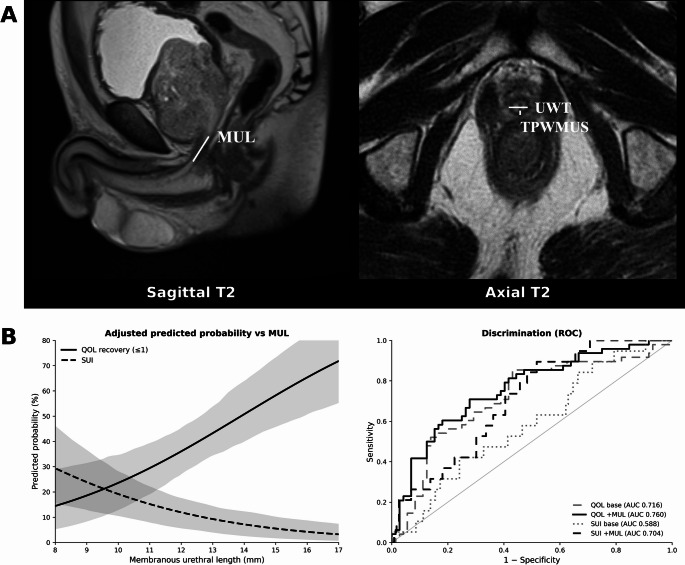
Preoperative membranous urethral length (MUL) and postoperative outcomes after HoLEP. A Representative MRI measurements. Left: MUL on a mid-sagittal T2-weighted image, measured from the prostatic apex to the bulbar urethra. Right: thickness of the posterior wall of the membranous urethral sphincter (TPWMUS) and urethral wall thickness (UWT) on an axial T2-weighted image at the membranous urethra. B Predicted probabilities and discrimination as a function of preoperative MUL. Left: adjusted predicted probability of 6-month IPSS-QOL recovery and stress urinary incontinence (SUI), with 95% confidence bands, with age and enucleated weight held at their means. Right: receiver operating characteristic (ROC) curves for the same outcomes, comparing the clinical base model (age + enucleated weight) with the MUL-based model. AUC, area under the curve
